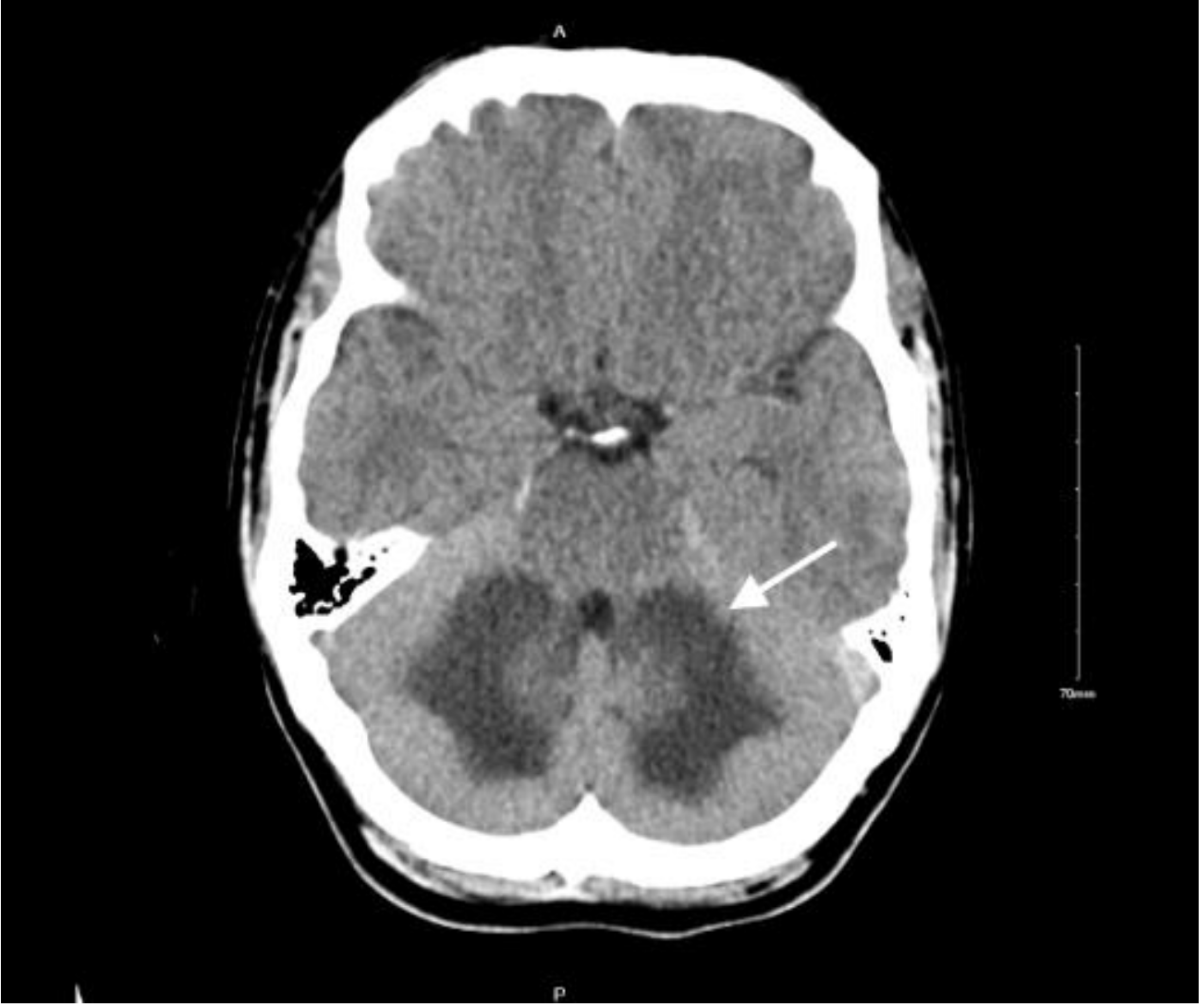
Axial head computed tomography (CT). Symmetric low attenuation lesions involving bilateral cerebellum measuring 5cm by 3cm are suspicious for acute to subacute infarct.
